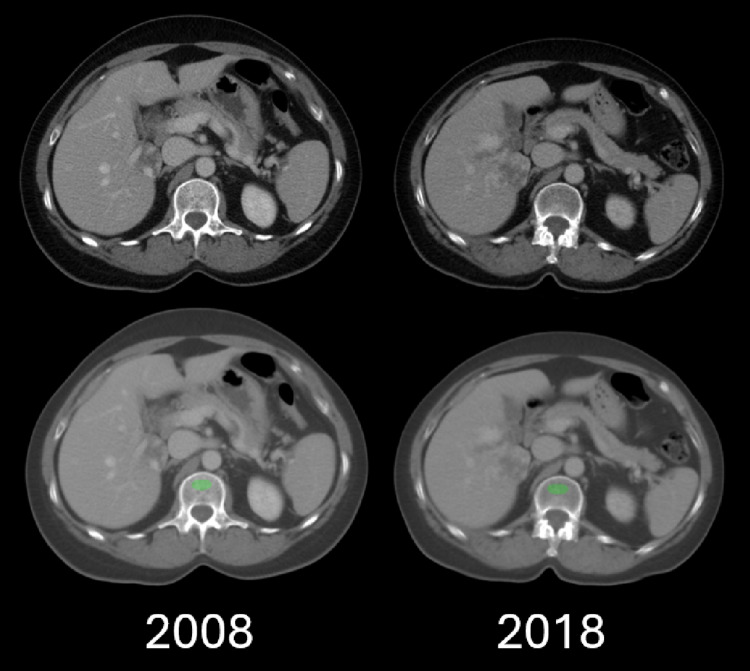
Change in L1 vertebral body attenuation over a 10-year interval. Change in L1 vertebral body attenuation in a 53-year-old female who underwent CT of abdomen with IV contrast media in 2008 (left column) and again in 2018 (right column) at age 64. Axial slices are shown at the L1 level for both time points, including original CT images (top row) and images showing the fully automated segmentation of L1 vertebral body trabecular bone (green). A decrease in L1 trabecular bone attenuation between 2008 and 2018 is visually apparent. AI-measured L1 vertebral body attenuation decreased from 166 Hounsfield units (HU) to 136 HU, for a net decrease of −30 HU over a 10.4-year interval and a rate of −2.9 HU/year. At both time points, the CT indication was to evaluate an incidentally discovered giant hemangioma, partially visible in the medial right lobe of the liver
